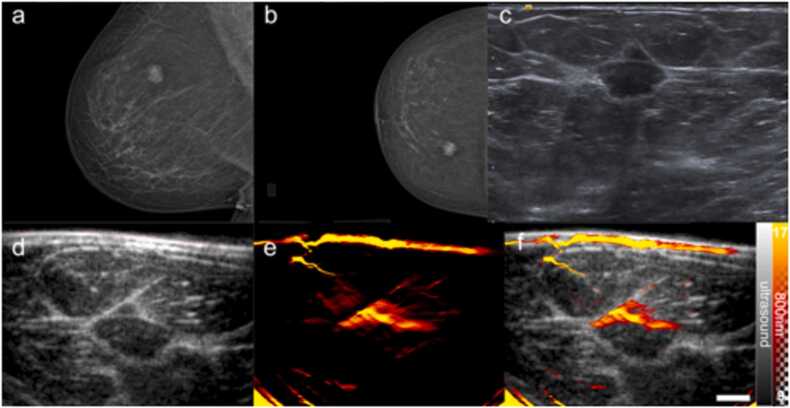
Exemplar grade 2 invasive ductal carcinoma (ER+ PR+ Her2-). Mammography [MLO (a) and CC (b) views] reveal a lobulated mass in the upper inner quadrant of the left breast. The outline has a few spicules. (c) Standard-of-care ultrasound reveals a hypoechoic irregular mass. (d) The US image from the OA-US scan also reveals an irregular hypoechoic mass, albeit at lower resolution than the clinical US imaging. (e) The OA image from the OA-US scan at 800 nm shows an irregular cap of signal at the anterior margin of the cancer (e) Overlaid OA-US image highlights the relationship between the visualised neoangiogenesis from OA and tumour mass position on US. Intense OA signals at the breast surface arise due to absorption in the skin by haemoglobin in the skin microvasculature as well as melanin pigmentation. Scale bar 1 cm.
